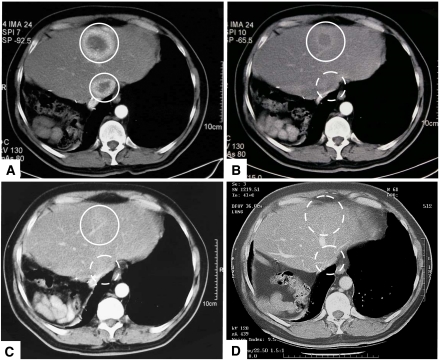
CT scans of the patient who showed sustained PR during the trial: At baseline (A), day 84 (B), 12 (C) and 36 (D) months post-treatment. Resolution of one liver metastasis is evident by day 84 with significant ablation of the second, which also eventually resolves. The patient is a long-term survivor at ++36 months and considered in full remission.
